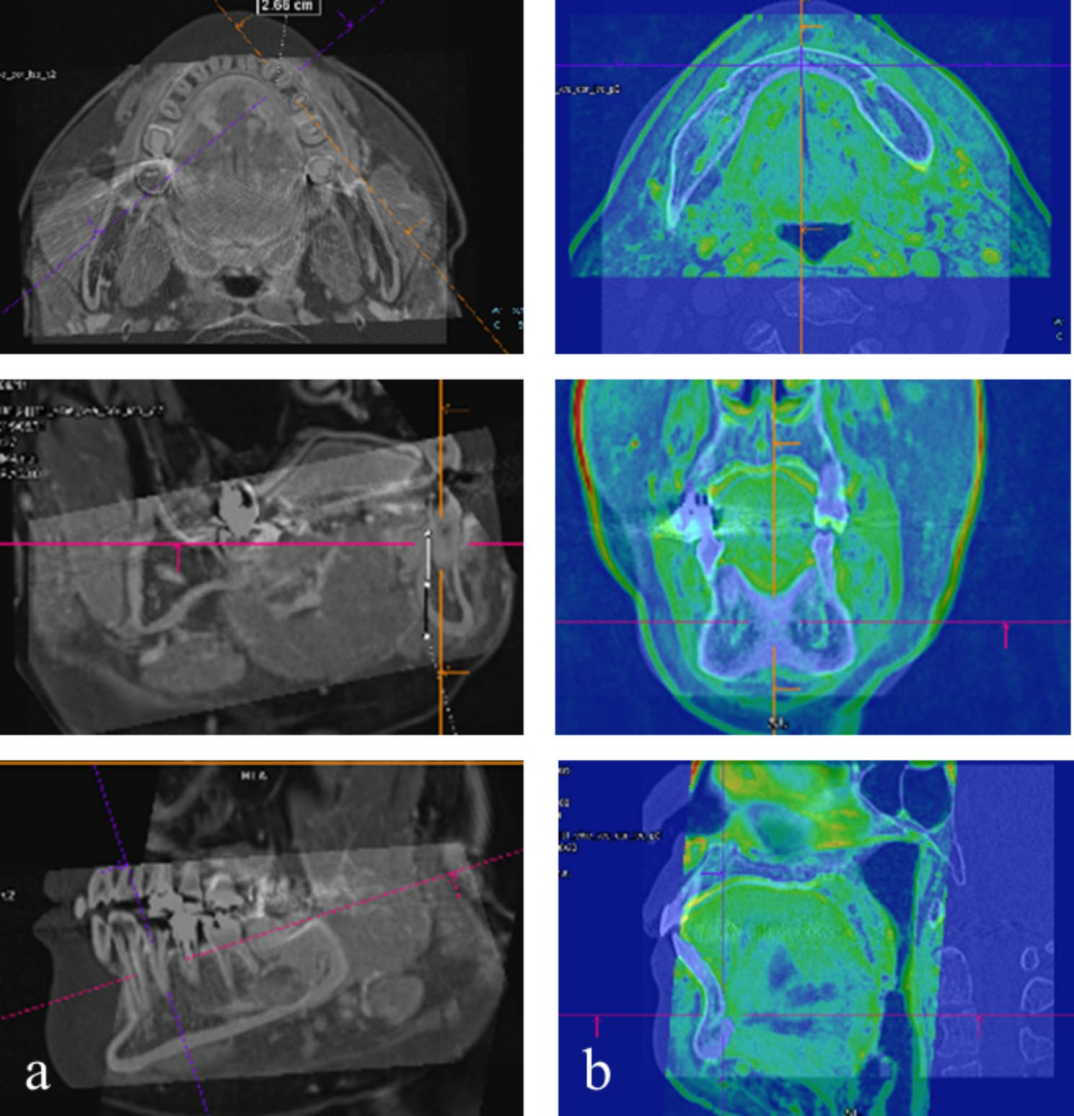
CT/MRI volumetric fusion images a. Fusion volumetric images before discoloration: CT/MRI volumetric fusion image was produced by two images of the mandible cortex completely overlapping b. Fusion volumetric images after discoloration
